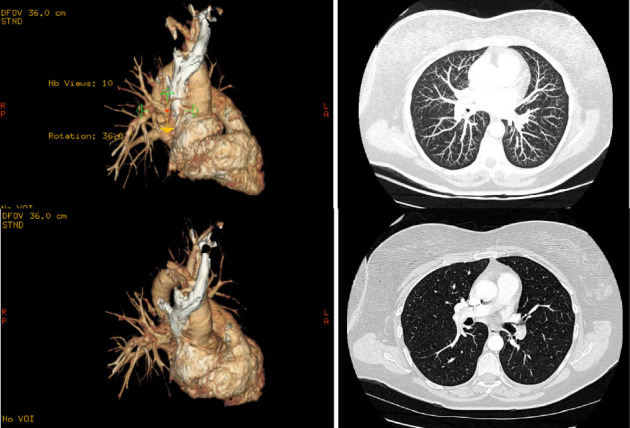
Volume rendering and axial imaging of initial diagnostic CT chest with angiogram protocol showing acute pulmonary sagittal embolus extending into the right and left pulmonary arteries and lobar, segmental, and subsegmental arterial branches bilaterally, with secondary right ventricular heart strain.
